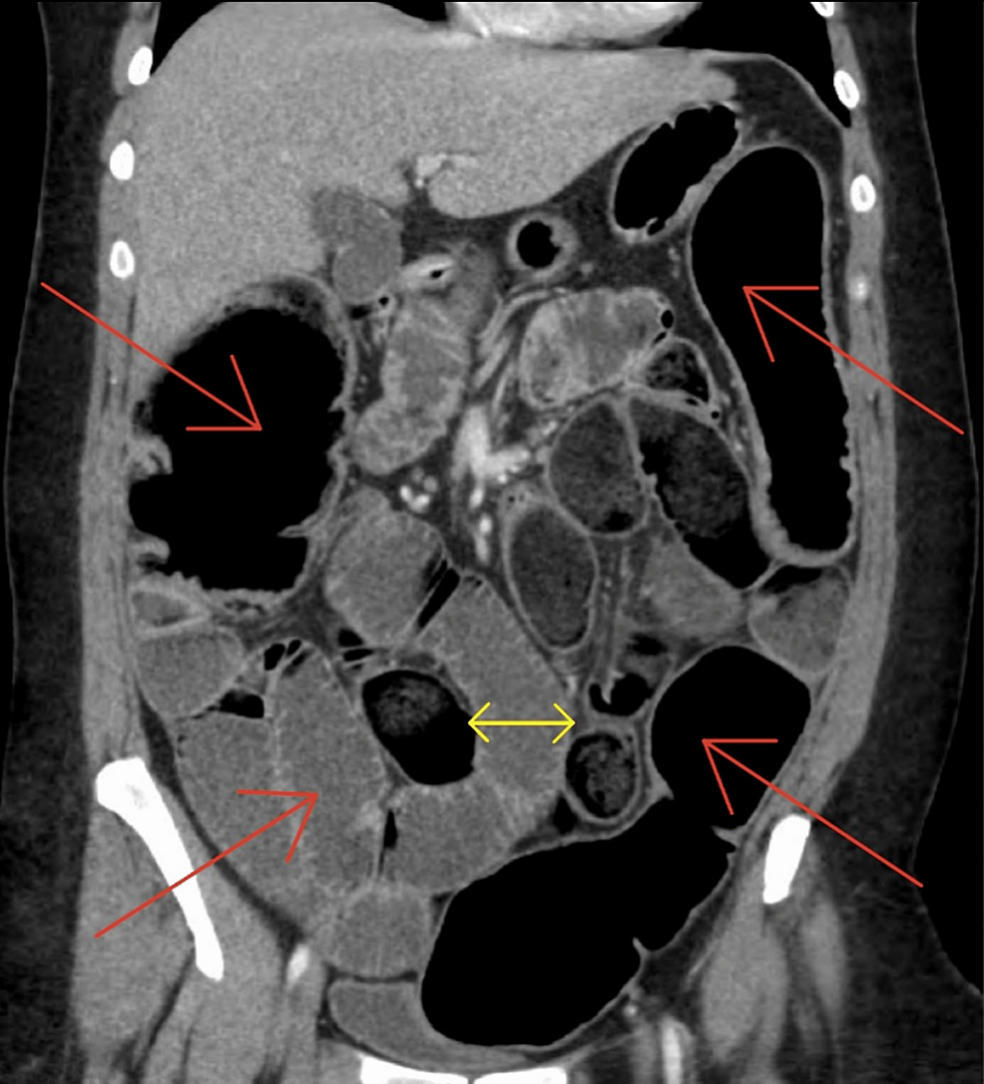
Coronal CT scan. Red arrows show severe distention of the small and large bowels. Yellow arrows show distention of the small bowel greater than 4 cm in diameter. CT, computed tomography
